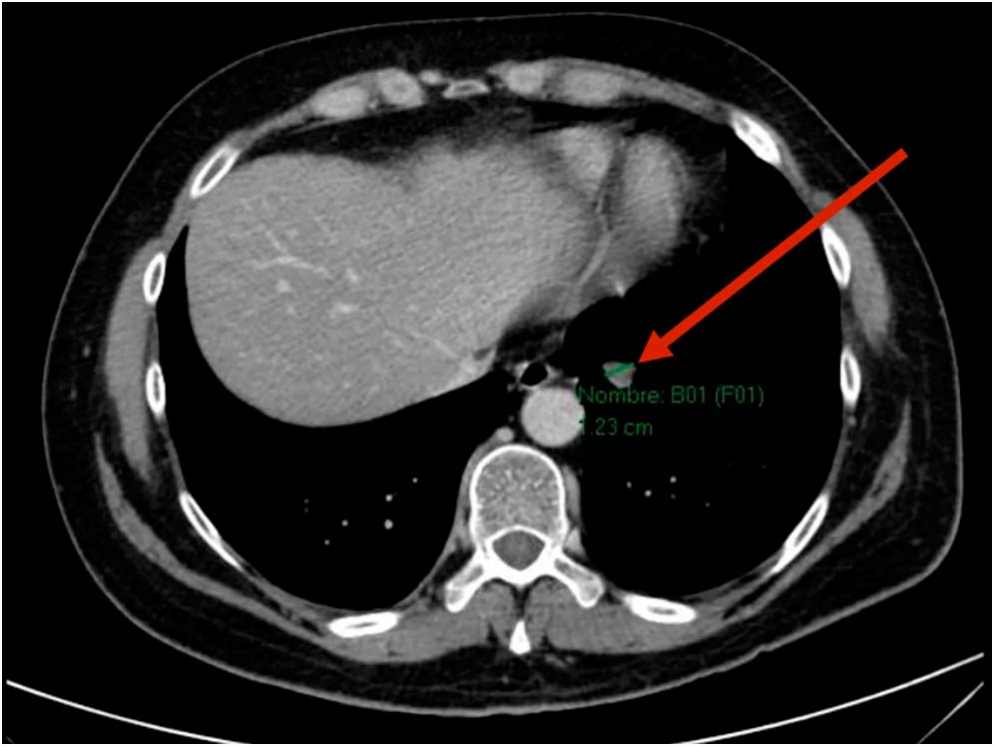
Thoraco-abdominal-pelvic CT scan. Axial view showing a 15 × 23 mm pulmonary nodular opacity at the base of the left lower lobe (arrow), without evidence of locoregional lymphadenopathy.
